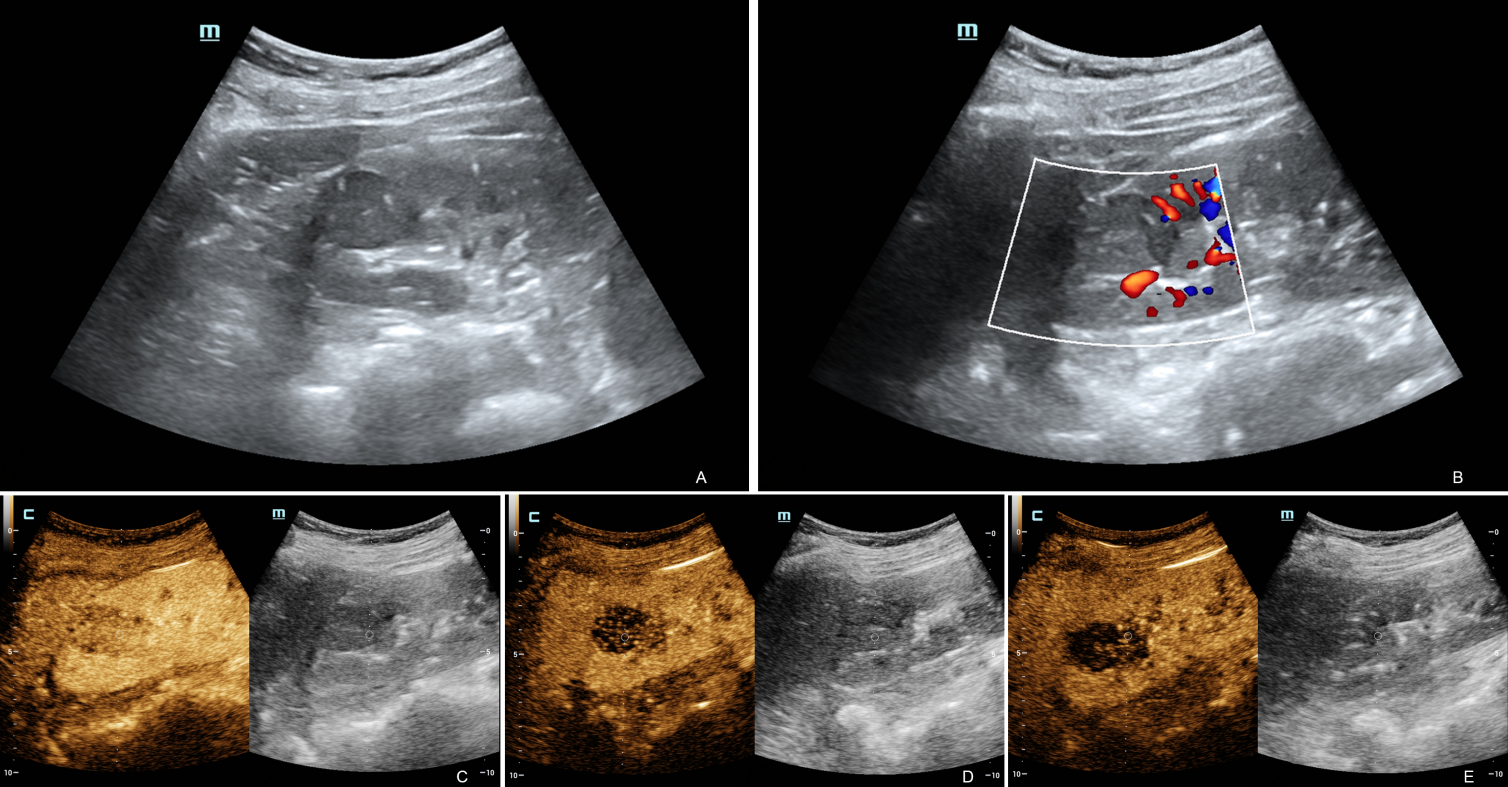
Ultrasound and contrast-enhanced ultrasound images of MTSCC. Ultrasound image showing a homogenous mass in the upper pole of the right kidney (A). Color Doppler ultrasound showed no obvious blood flow in the mass, but there was some around it (B). CEUS showed heterogeneous mild enhancement in the MTSCC lesion compared to the adjacent renal parenchyma in the cortical phase (C). In the medullary phase (D), the washout of the tumors was more simultaneous than that of the adjacent renal parenchyma. The lesion showed hypoenhancement during the delayed phase (E). These performances indicated hypovascular renal tumors.
